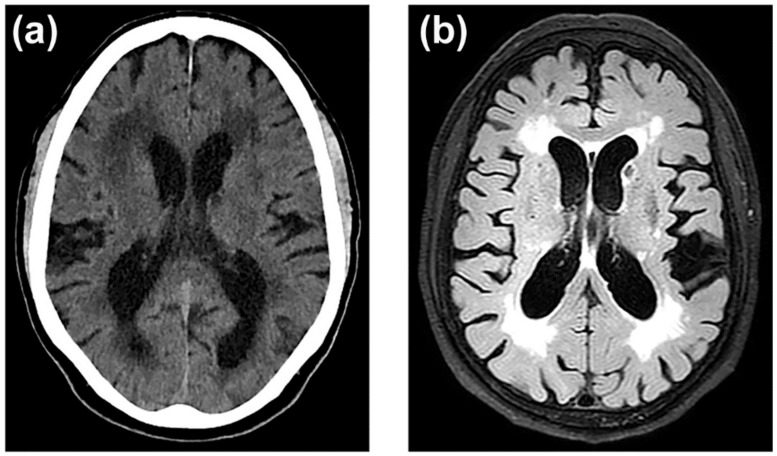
Leukoaraiosis in a 67-year-old hypertensive patient with occasional headaches. (a) CT showing diffuse hypodensity (rarefaction) of the periventricular white matter. (b) T2-MRI of the same patient, with sharper hypersignals.
